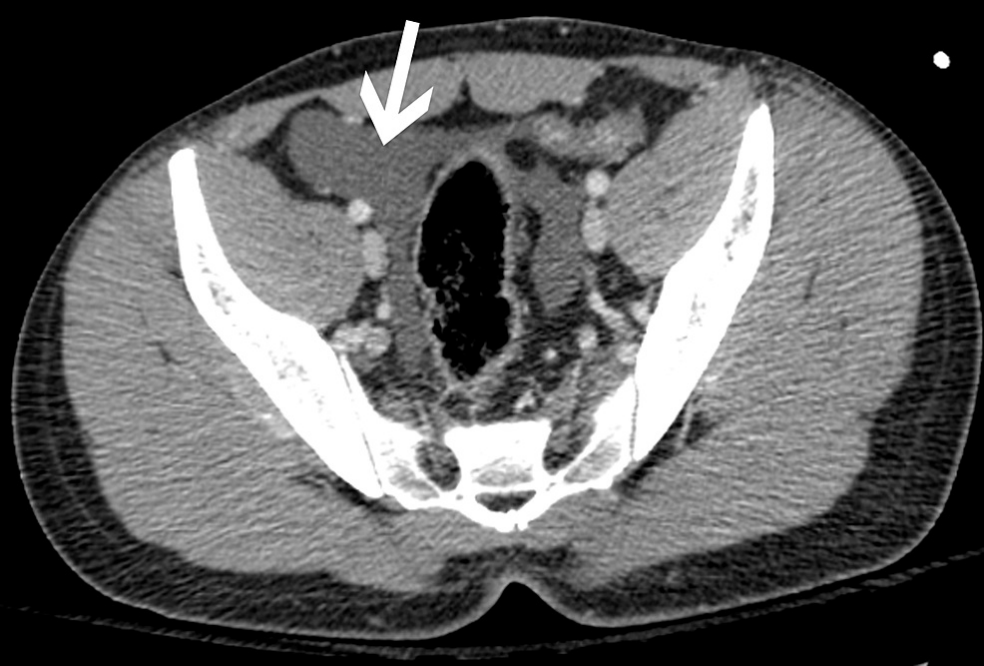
Axial CT image through the pelvis shows ascites (white arrow).
